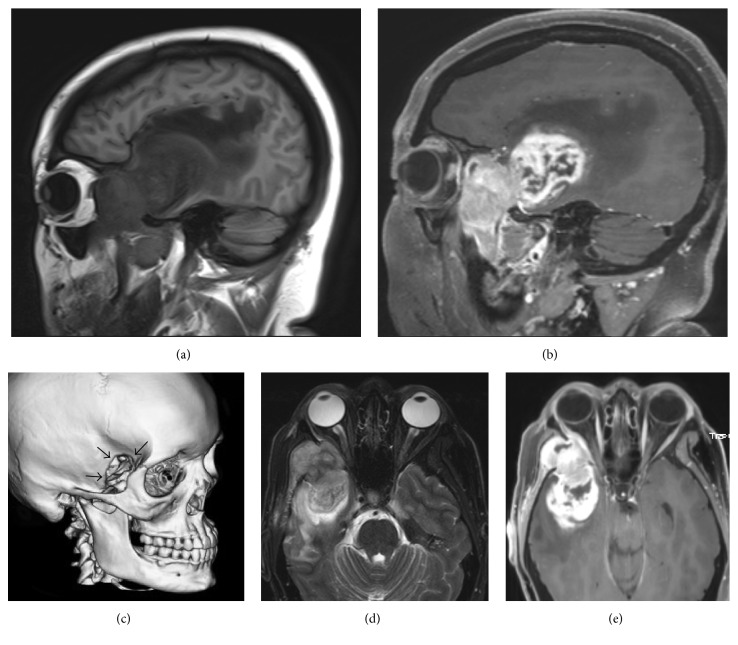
MRI and CT findings prior to surgery. (a) Sagittal T1-weighted imaging (T1WI) demonstrated a heterogeneous T1 hypointense mass involving a majority of the anterior temporal lobe with associated mass effect and surrounding vasogenic edema. Tumor extends through the greater wing of the sphenoid into the infratemporal fossa. (b) Sagittal postgadolinium T1WI demonstrates avid heterogeneous enhancement. The anterior portion in the soft tissues solidly enhances and intracranially has central necrosis. (c) 3D reconstruction from head CT demonstrates aggressive destruction of the greater wing of the sphenoid. (d) Axial FSE T2-weighted imaging demonstrates the mass to be heterogeneous iso/hypointense tumor with surrounding vasogenic edema suggesting increasing cellularity. (e) Axial postgadolinium T1WI demonstrates avid heterogeneous enhancement with central necrosis intracranially.
